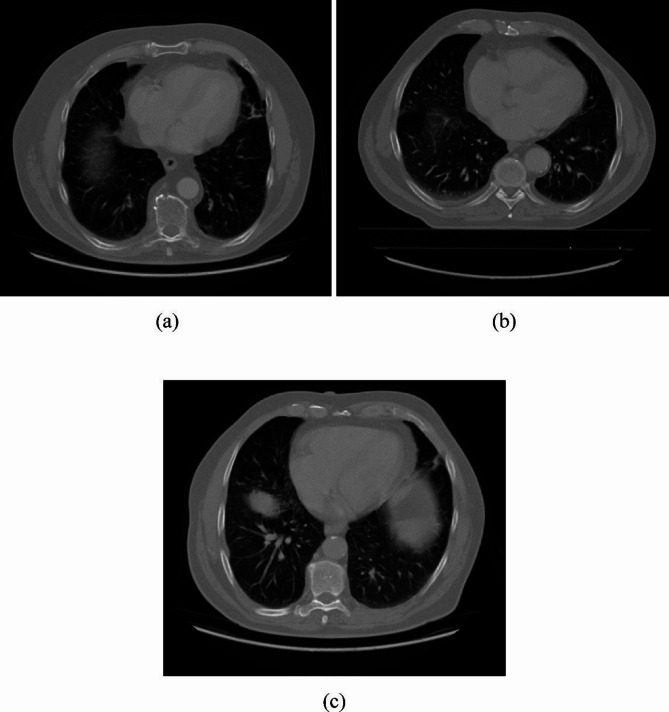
Representative CT slices (a) CT slice 1 (b) CT slice 2 (c) CT slice 3.
